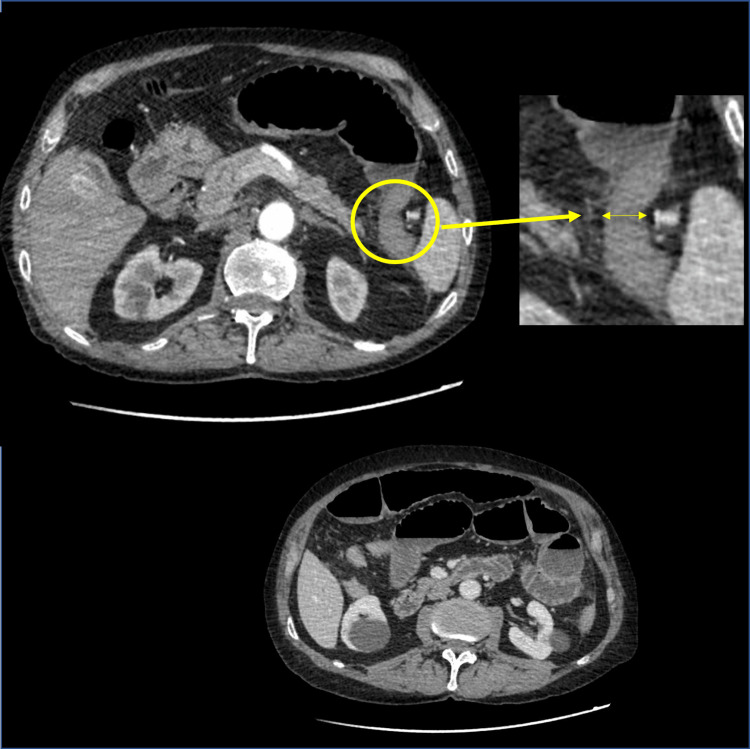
CT scan with a contrast-axial view A. Aspect of small bowel dilatation and depicting transverse colon-descending junction in relation to a dilated enteral loop (insert detail), without demarcation interface, in the left hypochondrium. B. Dilated bowel loops in the abdominal with horizontal hydro-aerial levels.
